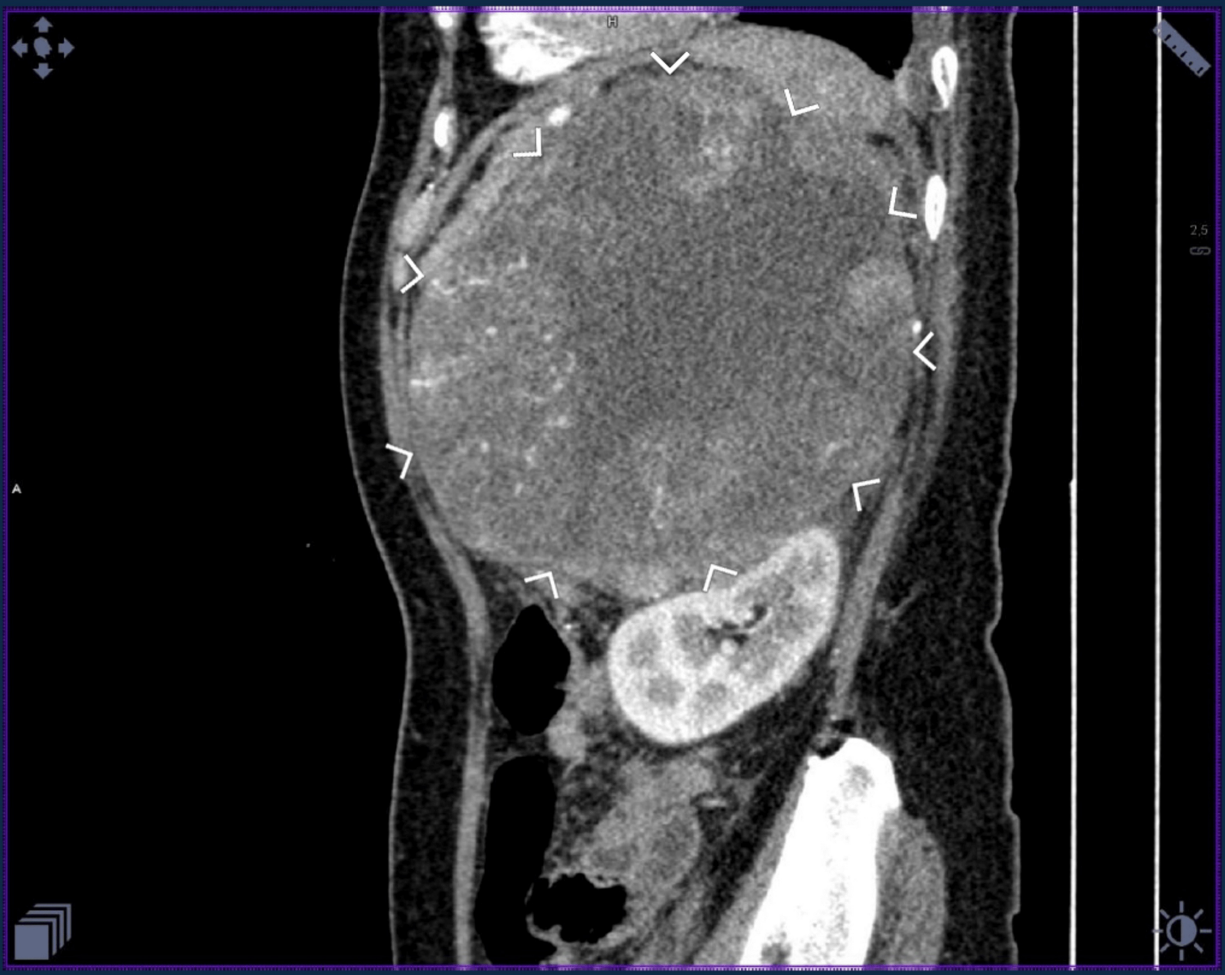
Computed tomography urogram, sagittal view, showing the large adrenal mass.
